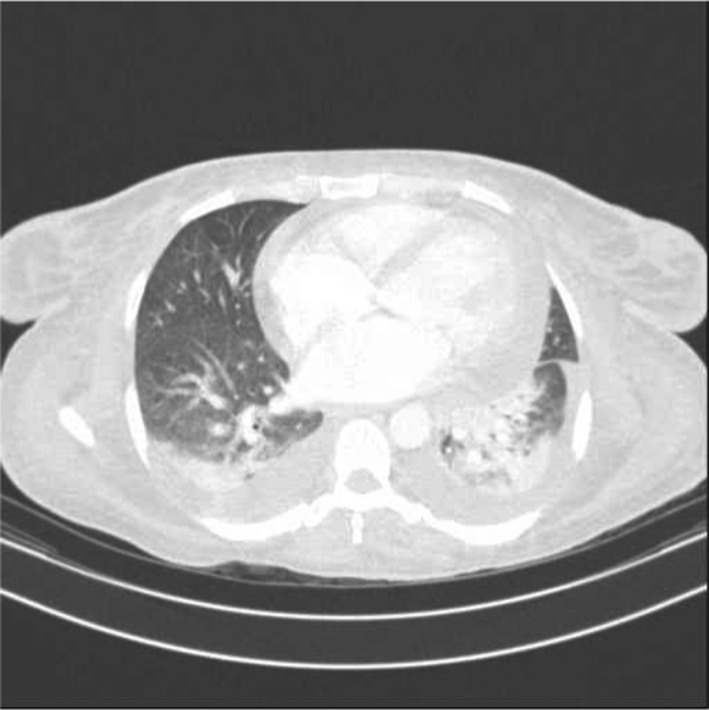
Axial thoracic CT scan cut of lower thoracic region set at pulmonary window; this cut depicts pleural effusion, ground‐glass opacity and collapse consolidation
